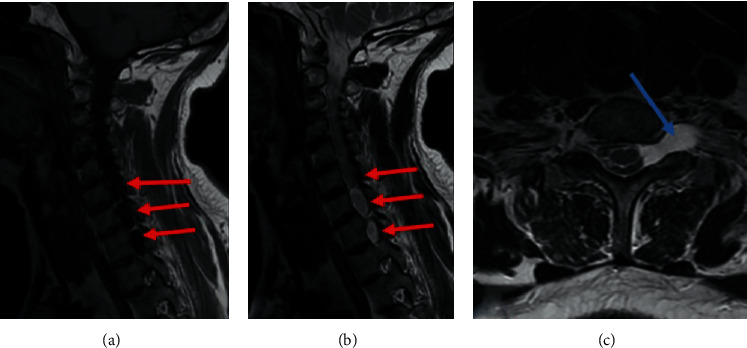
Cervical MRI, (a, b) T1 and T2 sagittal and (c) T2 axial sequences: pseudomeningoceles in the form of oblong formations of the C6-C7, C7-D1, and D1-D2 levels (red arrows) in T1 hyposignal, T2 hypersignal, extending into foraminal, and extraforaminal spaces (blue arrow). Note also the nonvisualization of the anterior and posterior rootlets, better visible on sagittal sections in favor of total avulsion.
